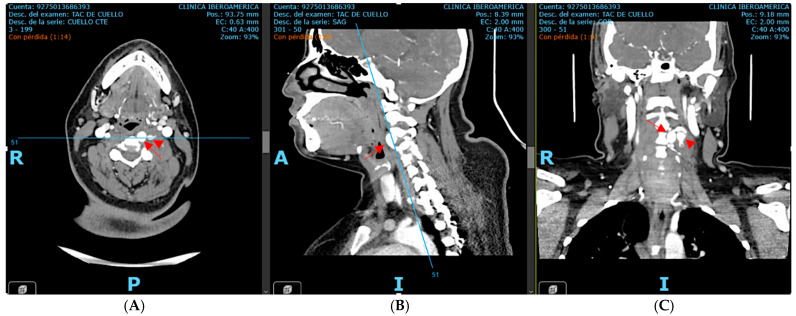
Contrast-enhanced CT angiography of the neck and cerebral vessels, axial view (A), sagittal view (B), and coronal view (C), performed after intravenous administration of 100 mL of non-ionic iodinated contrast medium (Iohexol, Omnipaque^®^ 300 mg I/mL, injection rate 3–4 mL/s). Image acquisition was performed during the arterial phase using a bolus-tracking technique (approximately 20–25 s after injection, triggered when the contrast density in the aortic arch reached ≥120 Hounsfield units). The study demonstrates a tortuous left vertebral artery with fusiform aneurysmal dilation of the V2 segment, measuring approximately 10 × 14 mm. A focal mural defect is identified, with active contrast extravasation into a contained perivertebral hypodense collection (34 × 24 mm). The aneurysmal dilation is marked by the red arrow, while the mural defect is indicated by the arrowhead. Source: patient’s medical record, published with prior authorization.
